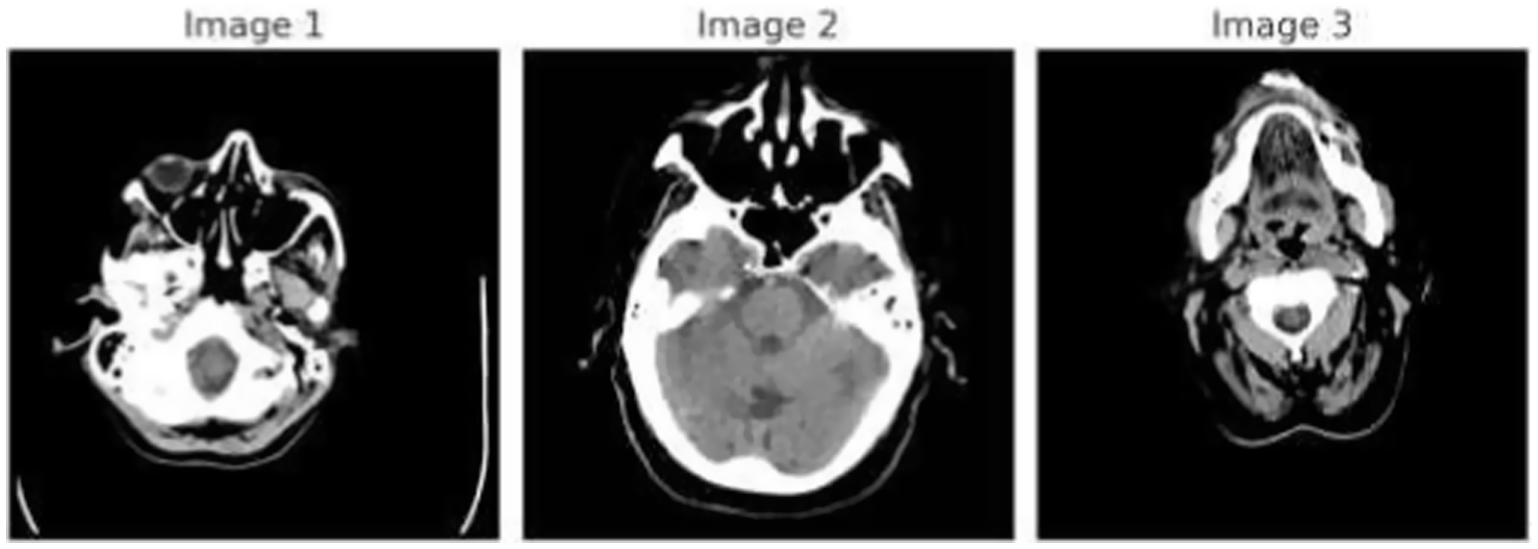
Dataset overview: raw and processed brain CT scan images.
